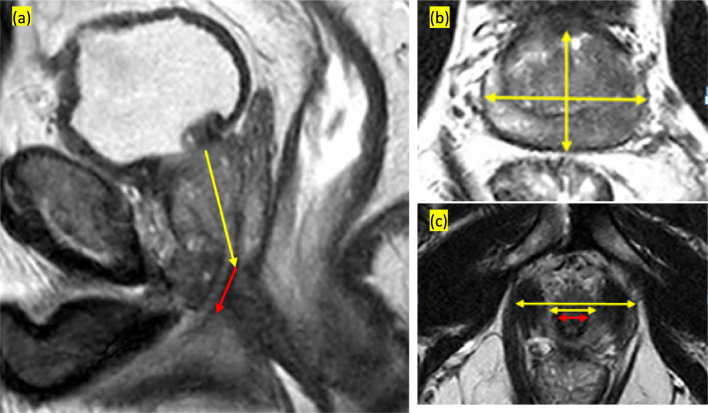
a Midsagittal T2-weighted image. The yellow arrow displays maximum height of the prostate, measured from base to apex. The red arrow defines MUL, measured from the apex of the prostate to the upper border of penile bulb, at the dorsal side of the urethral lumen. b Axial T2-weighted image at maximal prostate width. The horizontal yellow double arrow shows maximal prostate width and the vertical yellow double arrow displays maximal prostate length. c Axial T2-weighted image immediately below the caudal margin of prostate apex. The horizontal red double arrow represents urethral width. The shorter and the longer yellow double arrows display the ILD and OLD, respectively. *ILD* inner levator ani muscle distance, *MUL* membranous urethral length, *OLD* outer levator ani muscle distance
